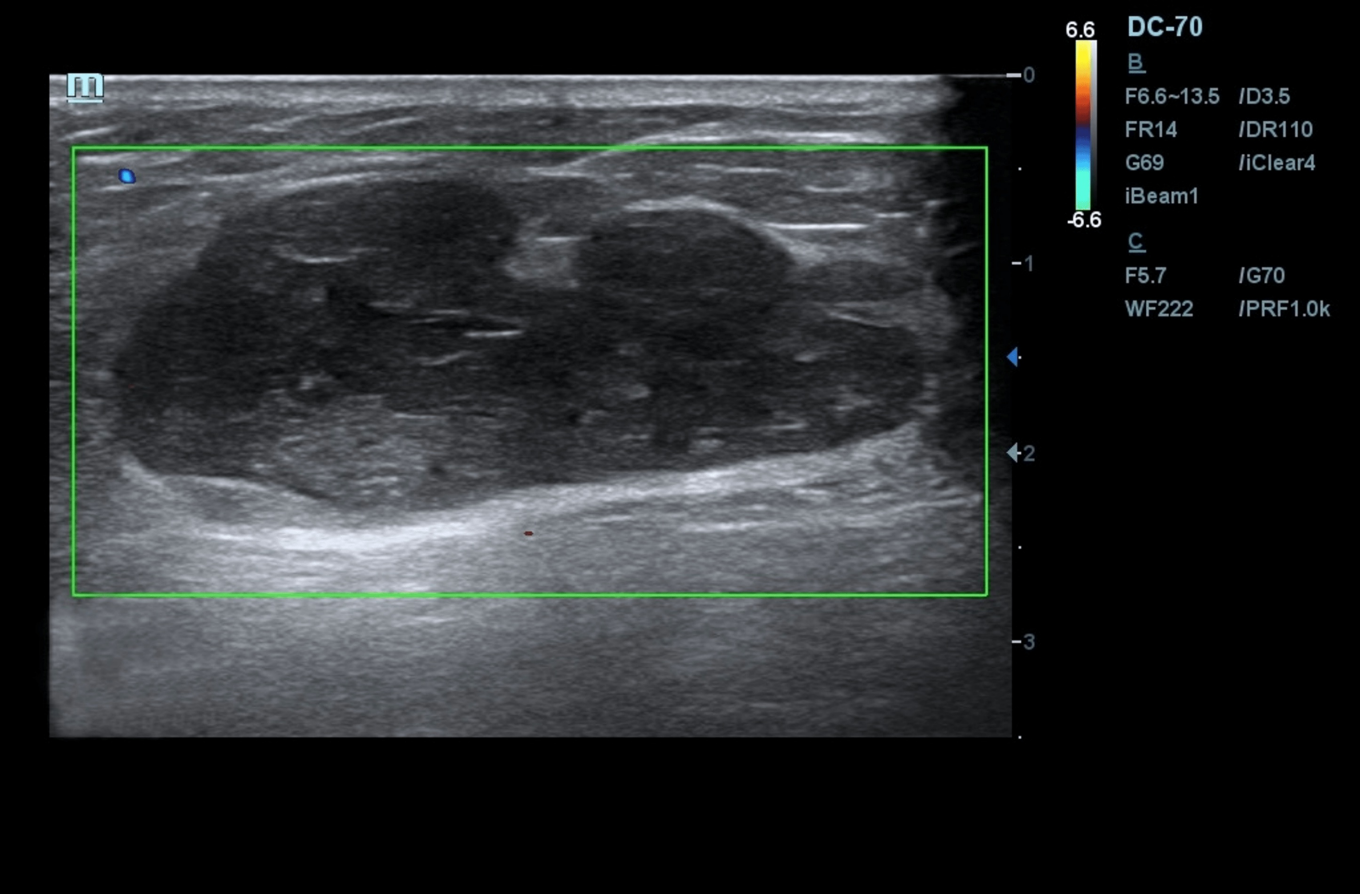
Sonogram of patient S in standard B-mode. Performed in two standard projections: in the central part of the left breast, an encapsulated volumetric nodular neoplasm of irregular oval shape of heterogeneous echostructure with anechogenic and hyperechogenic inclusions of BIRADS 4c category can be visualized.
